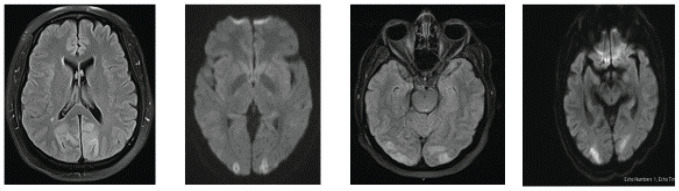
MRI brain of 1 to 4th case from left to right showing PRES.
